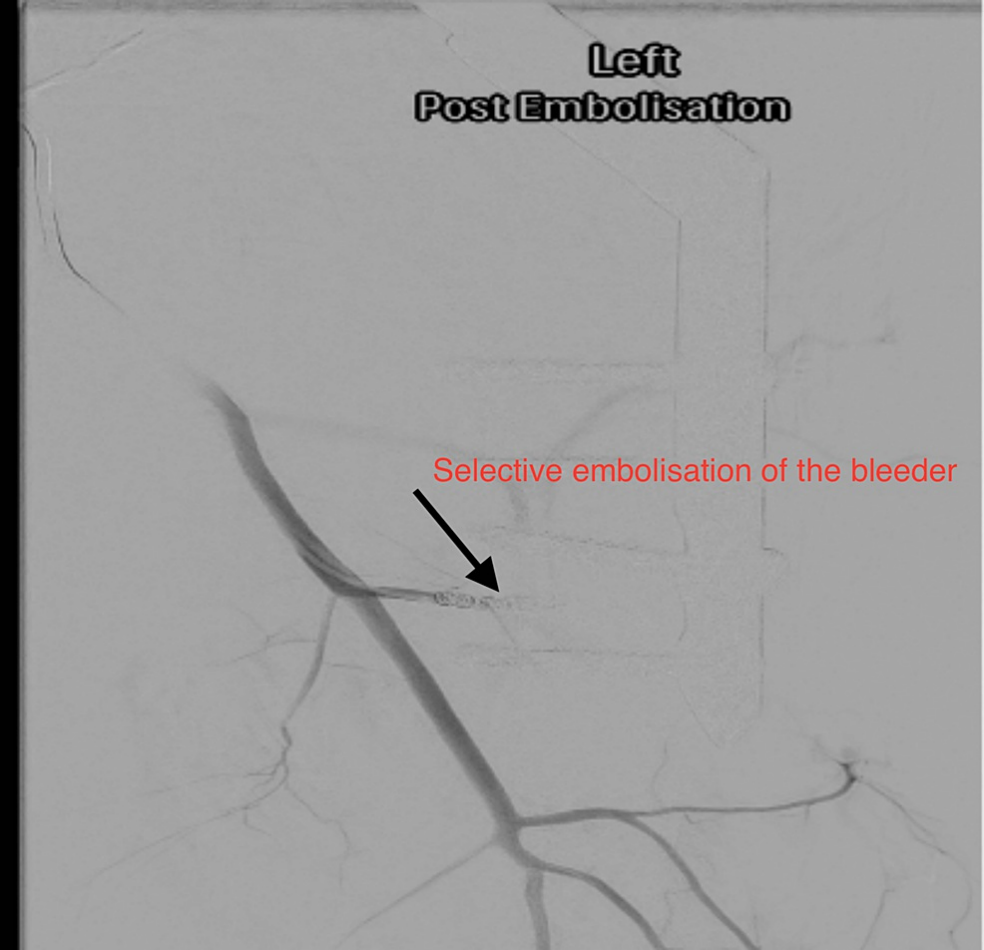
Post-embolisation images on CT angiogram.
